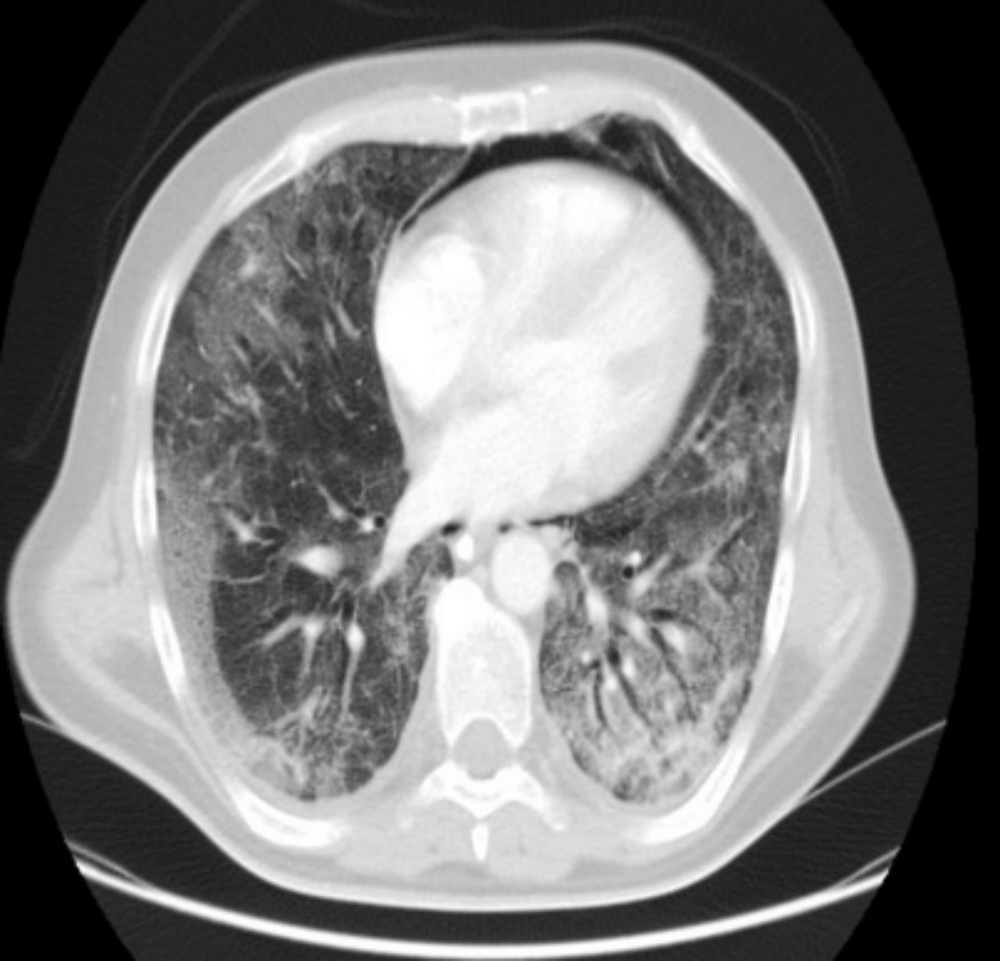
CT scan at hospital admission CT scan showing bilateral ground glass opacities and crazy-paving pattern
